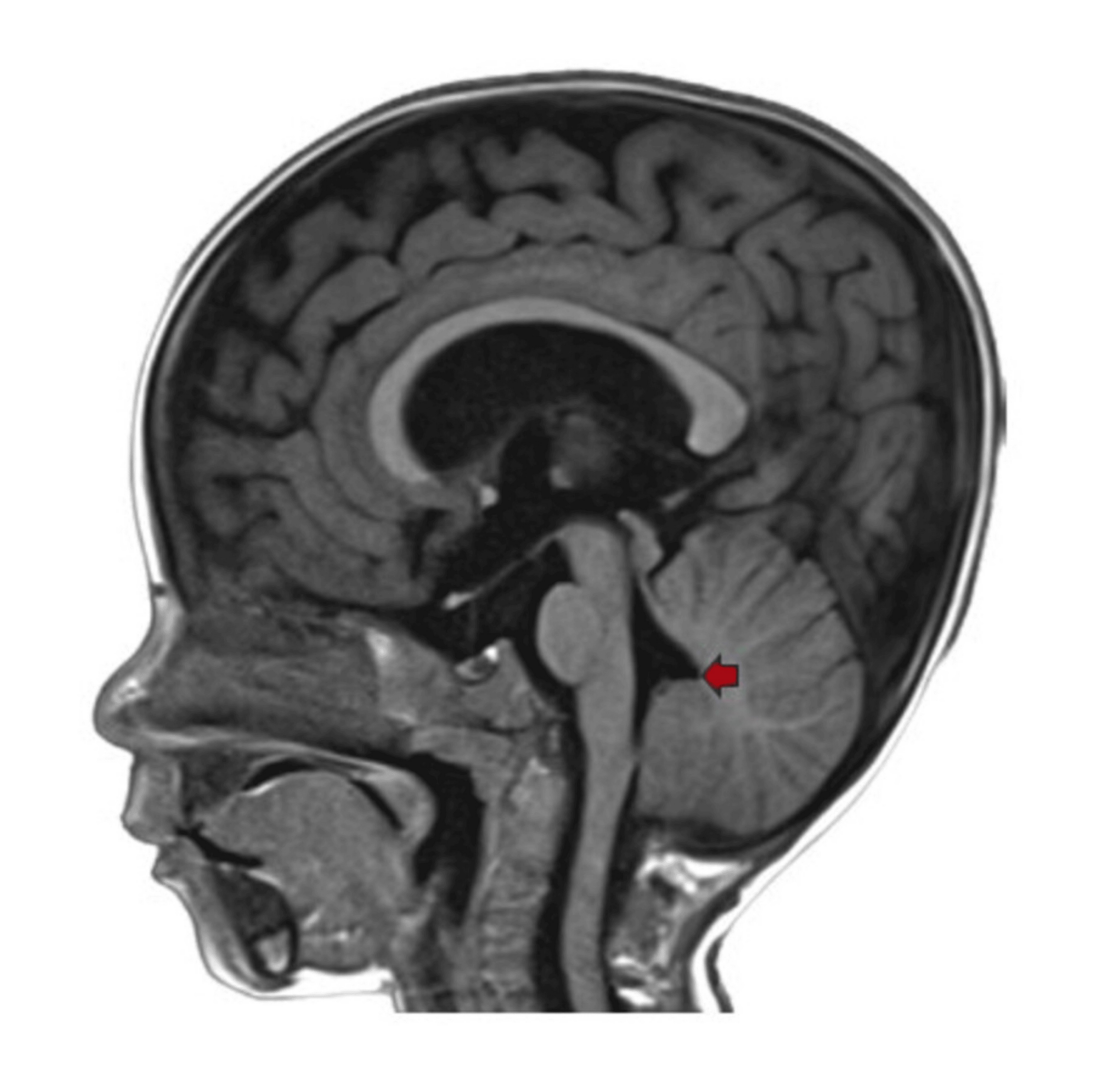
MRI T1WI sagittal image The image depicts the absence of the primary fissure and rounding of the fastigial recess (red arrow) MRI: magnetic resonance imaging; T1WI: T1-weighted imaging
